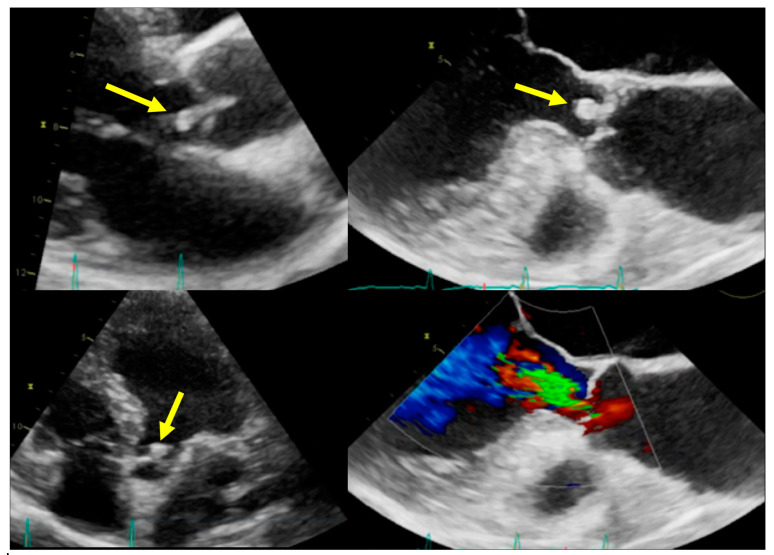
Infective endocarditis (IE) on aortic valve. Top and bottom left: Parasternal long axis and apical four-chamber transthoracic echocardiography (TTE) showing vegetation (arrows) in the left ventricle outflow tract. Top and bottom right: Transoesophageal echocardiography (TOE) showing the vegetation and the related valvular regurgitation.
